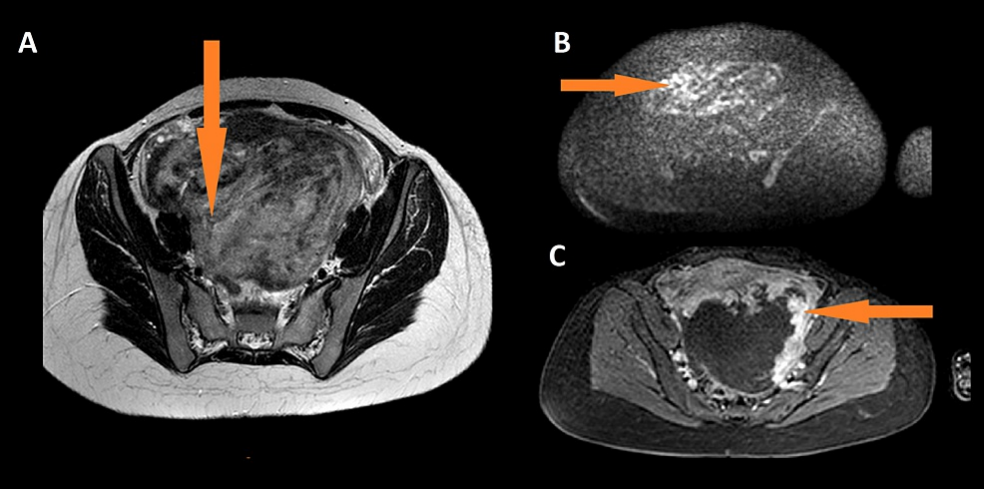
Axial T2(A), axial diffusion(B), and axial T2 C+(C) magnetic resonance imaging (arrow) Showing heterogeneous signal on T2 with hyperintense signal on diffusion, and intense peripheral enhancement.
